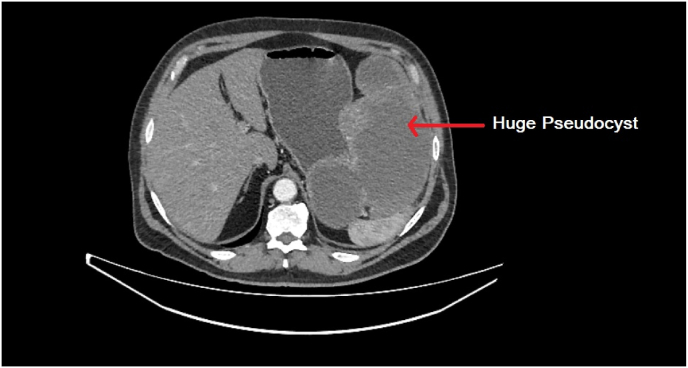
CT scan of the abdomen revealing a huge pancreatic pseudocyst.
